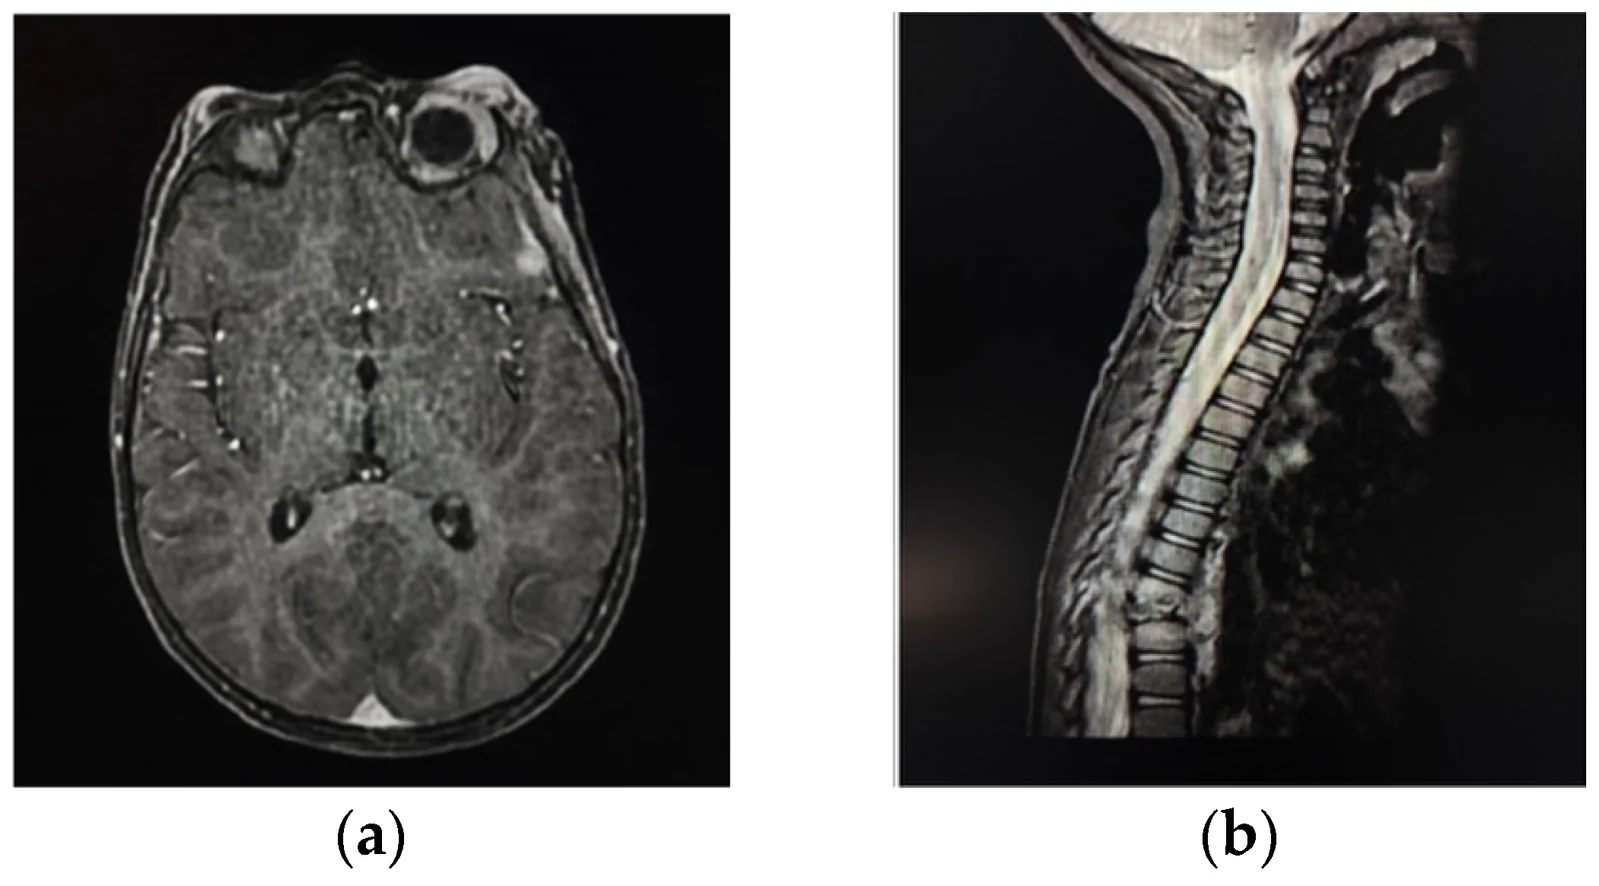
MRI of the brain and spine: (a) a hyperintense lesion in the left frontal region; (b) erosion and destruction of the D10 vertebral body with involvement of the adjacent intervertebral disc and an associated paravertebral abscess.
